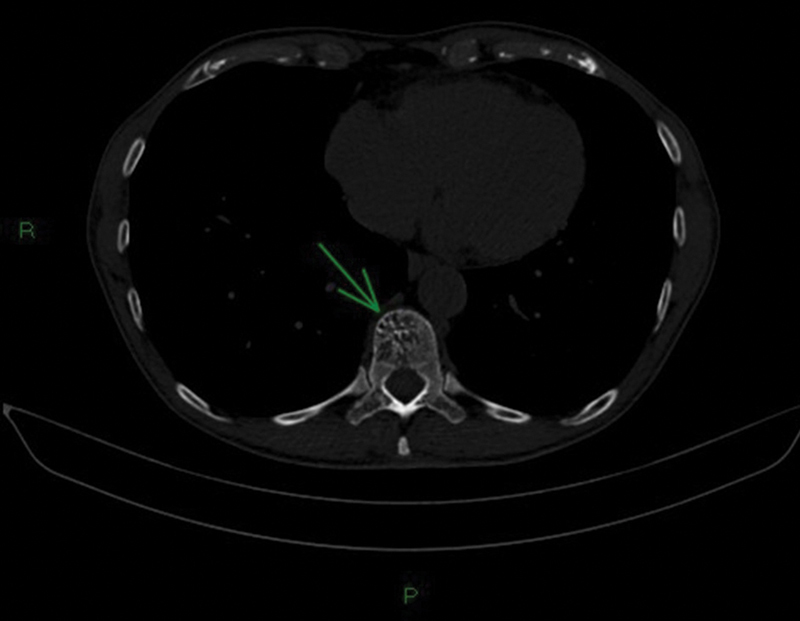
Axial slice image showing the so-called “polka-dot” or “salt and pepper” sign in which cross-section of the vertebra on computed tomography shows foci of high density owing to trabecular thickening, a finding characteristic of hemangiomas (green arrow).
